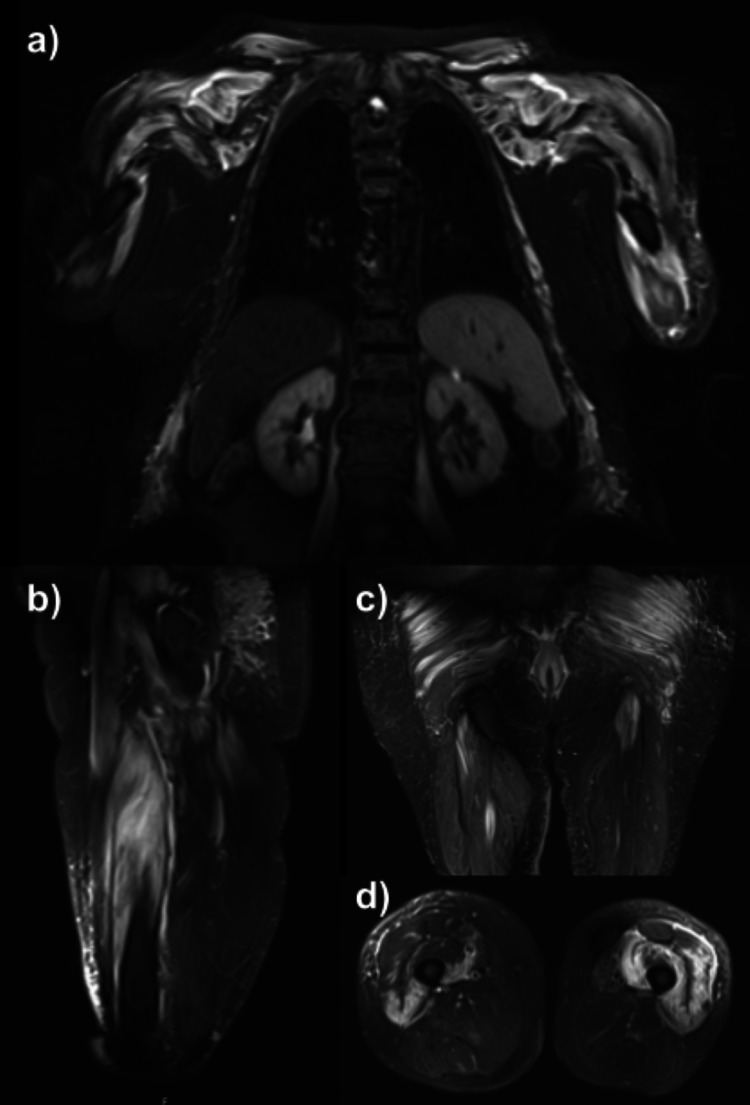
MRI - T2-weighted images presenting hyperintense signal throughout the affected muscles and peri-muscular oedema (a) Periscapular muscles in coronal view. (b) Thigh muscles in sagittal view. (c) Hip muscles in coronal view - gluteal aspect. (d) Thigh muscles in axial view.
